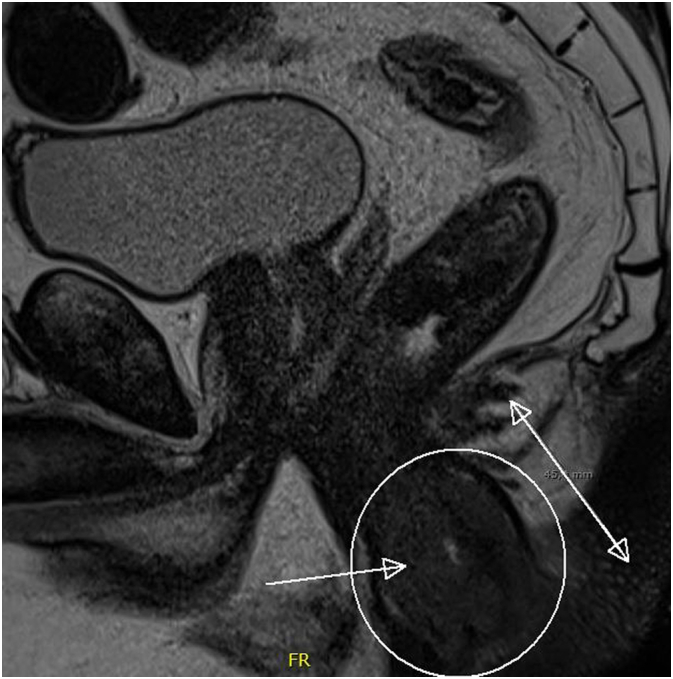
MRI scan at recurrence shows a mass in the anal canal bulging out of the anus.
